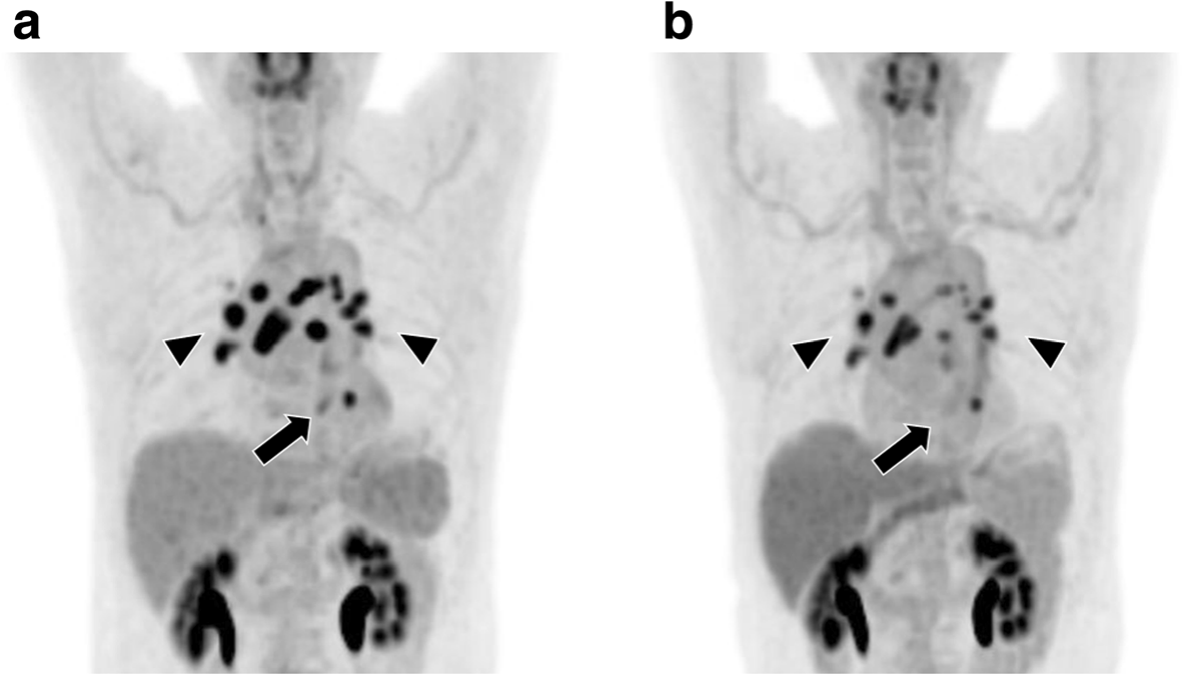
FDG-PET/CT images obtained before (a) and after (b) chemotherapy in case 1. a Images obtained before chemotherapy show FDG accumulation in the primary cancer (arrow; SUV max: 4.06) and in the hilar-mediastinal lymph nodes (arrow head; SUV max: 15.0). b Images obtained after chemotherapy show a disappearance in FDG uptake in the primary cancer (arrow) and a slight reduction in FDG uptake in the hilar-mediastinal lymph nodes (arrow head; SUV max: 8.07)
